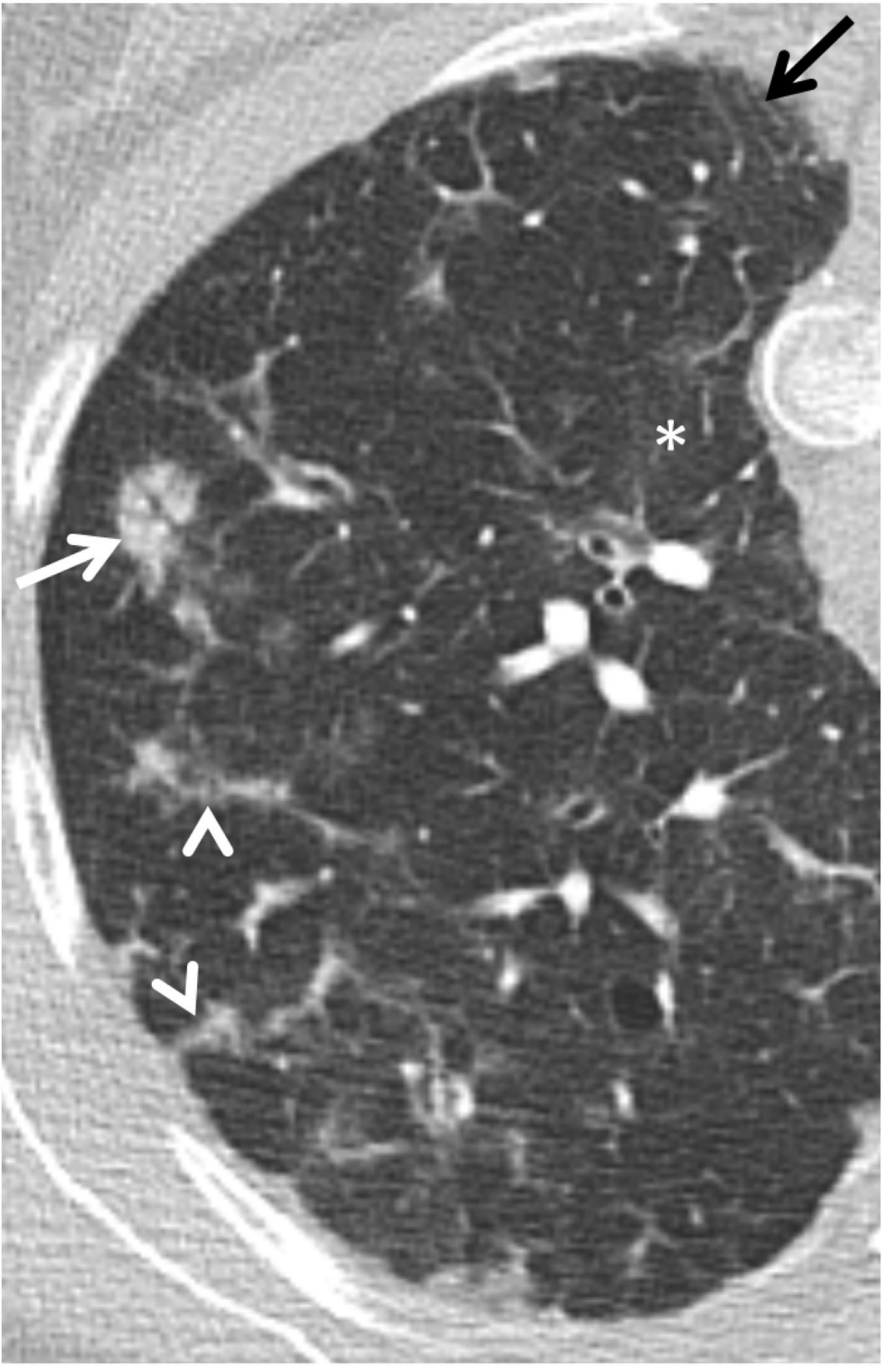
HRCT image of the right upper lobe of a 40-year-old woman with characteristic findings of granulomatous-lymphocytic interstitial lung disease (GLILD) with irregular peribronchovascular interstitial thickening (white arrow), interlobular septal thickening (arrowheads), subtle ground glass opacities (asterix), and traction bronchiectasis (black arrow).
